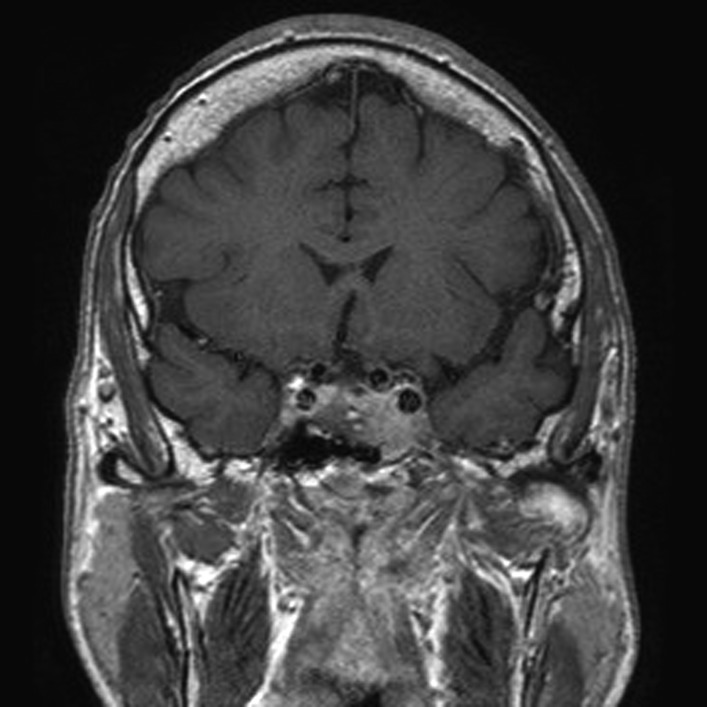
Before pasireotide therapy. MRI scan of pituitary – coronal view – T1 weighted image with gadolinium contrast showing residual pituitary tumour with invasion of cavernous sinus showing homogenous enhancement.
